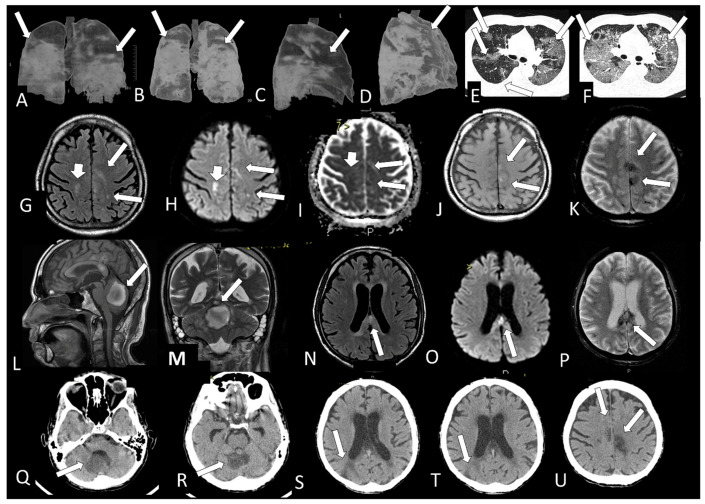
Case 31—The Chest CT on admission shows areas of alveoellar consolidation and ground glass in more than 50% of lung area (arrows A,C,E). Three days later, there was a severe increase of the lung disease (arrows, B,D,F). The brain MRI shows some hyperintense small areas on FLAIR (arrows, G) and in DWI (arrows, H) which are hypointense on ADC-Map (restricted diffusion lesions, arrows, I) in both cerebral hemispheres. Small areas of microbleeding are observed, being hyperintense on T1WI (J, arrows) and hypointense on T2* (K, arrows) localized on the medial part of left pre and postcentral gyrus. There is also a large oval hematoma located at the vermis of the cerebellum, being hyperintense on sagittal T1 (L, arrow) and on coronal T2WI (M, arrow) with peripheric hypointense ring of hemosiderin. Some small lesions, which represent microbleeding (methemoglobin), are also located on the splenium of the corpus callosum hyperintense on FLAIR (N, arrow) and on DWI (O, arrow) being hypointense on T2* (P, arrow). The CT (Q–U) without contrast made one month later showed that the lesions described above on previous MRI are hypointense and that a new sequel in the right parietal lobe (S,T, arrow) had appeared.
